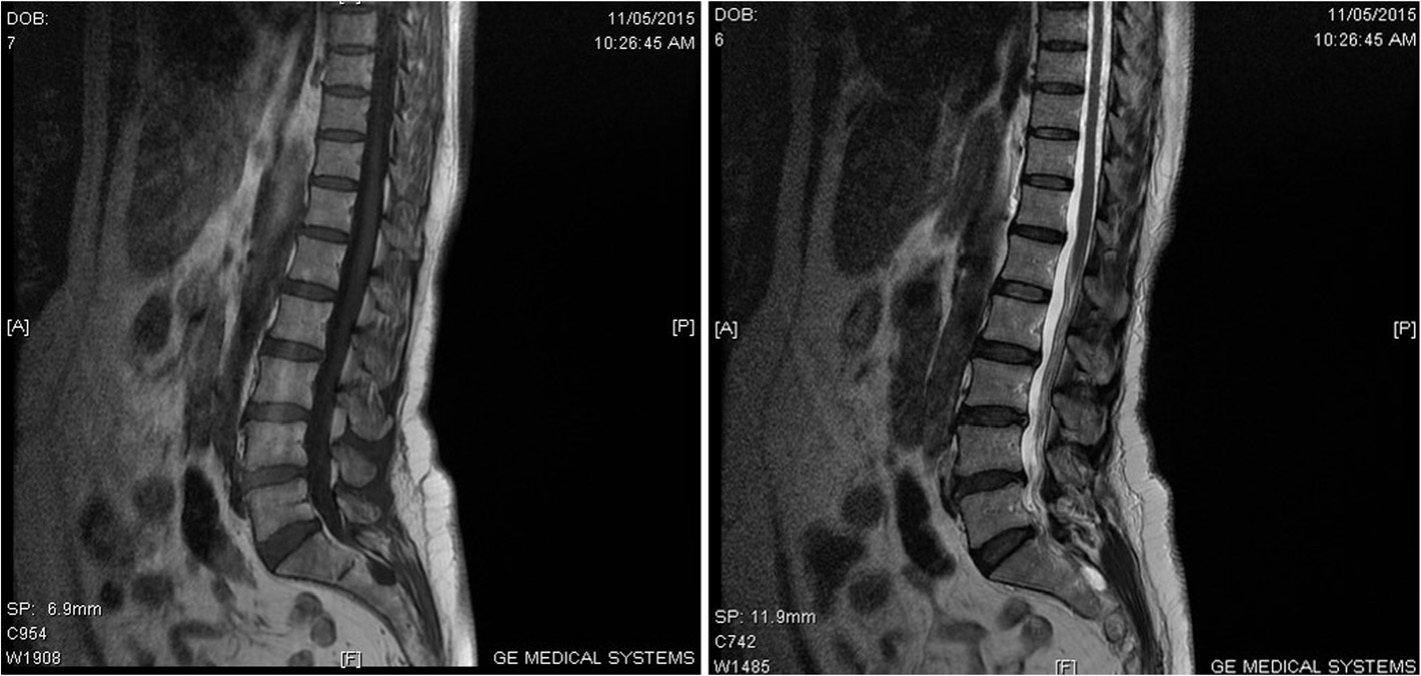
Lumbosacral magnetic resonance imaging scan showing a sacral Tarlov cyst (T1 and T2)
